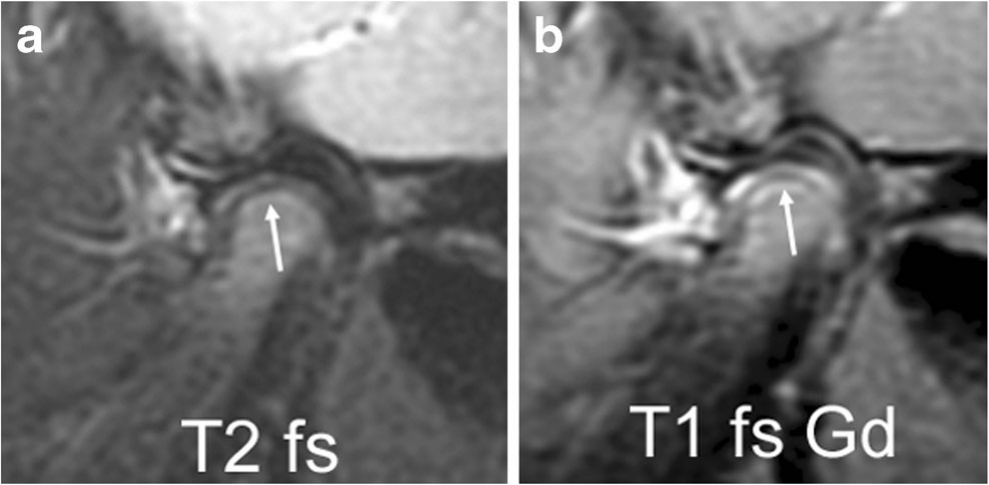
Mandibular growth zone. Sagittal oblique fast spin echo images in a 3-year-old girl show the mandibular growth zone at the surface of the condyle. a Beneath the low signal intensity line of cartilage and subchondral bone, this is seen as a narrow zone of high signal intensity (arrow) on fluid-sensitive sequence (TR/TE 5,400/77 ms, fat-saturated). b On postcontrast fat-saturated T1-weighted images (TR/TE 670/10 ms) there is corresponding enhancement. These findings represent the well vascularised zone of endochondral ossification. Note enhancement of the whole lower joint compartment as well as anterior recess of upper compartment. T1 fs Gd contrast-enhanced fat saturated T1-weighted, T2 fs fat saturated T2-weighted
